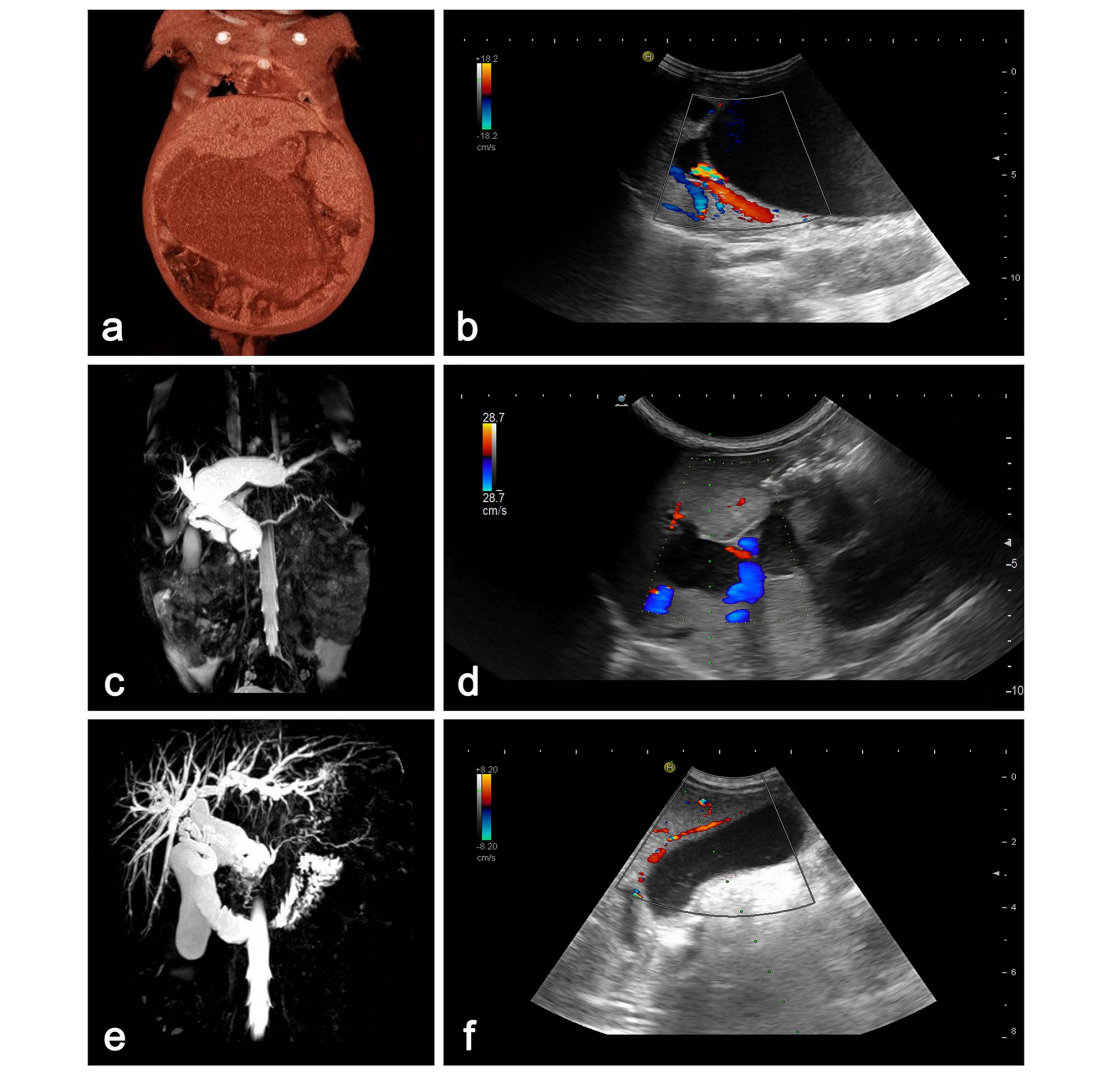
CT or MRCP, and US results of patients with choledochal cyst before and during US-guided percutaneous external drainage. (A) CT before US-guided PBD, Case 1 with a giant choledochal cyst. (B) US-guided PBD, Case 1 with a giant choledochal cyst. (C) MRCP before US-guided PTCD, Case 5 with significant dilatation of the intrahepatic bile duct. (D) US-guided PTCD, Case 5 with significant dilatation of the intrahepatic bile duct. (E) MRCP before US-guided PTGD, Case 6 with a large gallbladder. (F) US-guided PTGD, Case 6 with a large gallbladder
